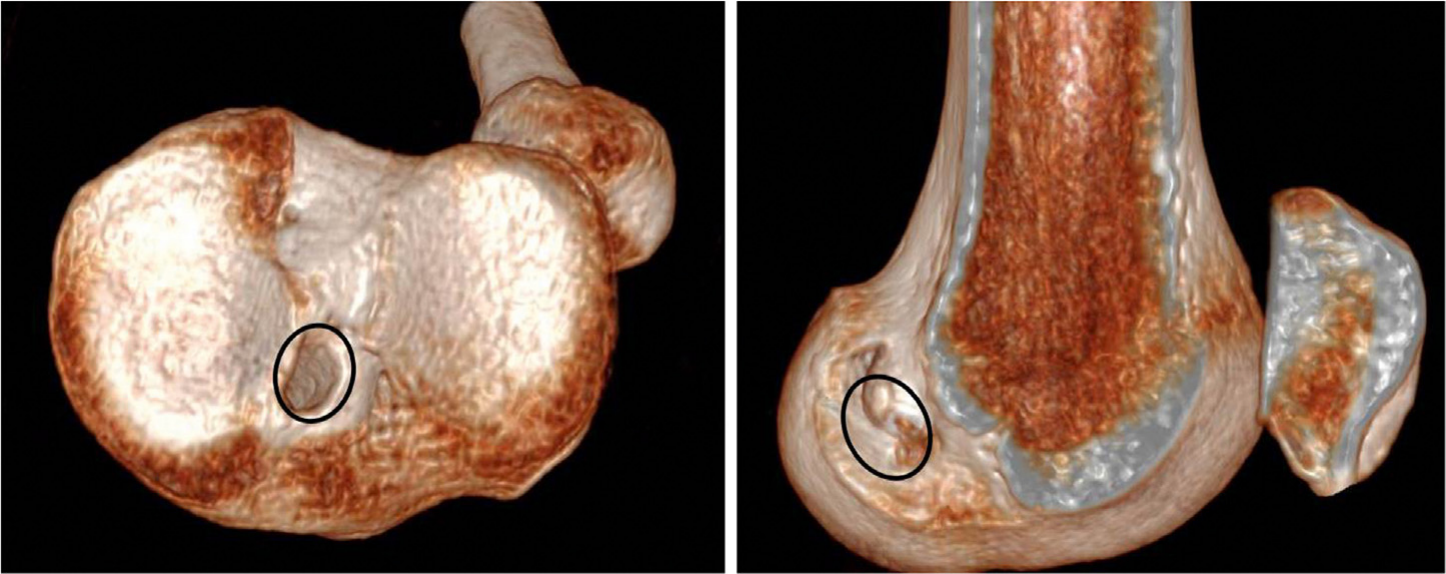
Three-dimensional computed tomography images of a left knee showing aperture locations of the tibia tunnel and femoral socket (black ovals). In this case, both seem appropriately located.3.The axial CT cut confirms over 5 mm deep persistent distal patella bone defect (Fig 3).
